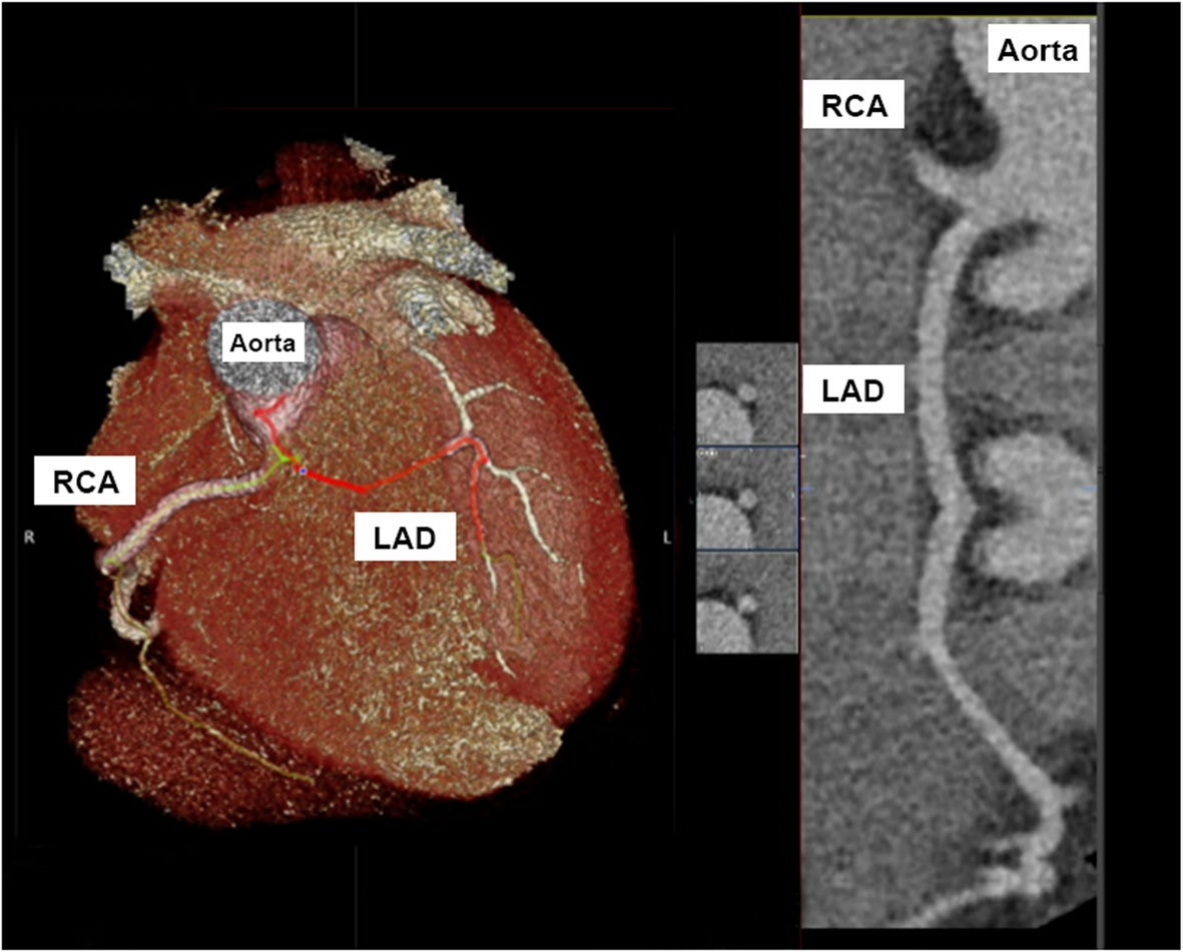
Rendered 3D Computed Tomography showing course of LMCA/LAD
